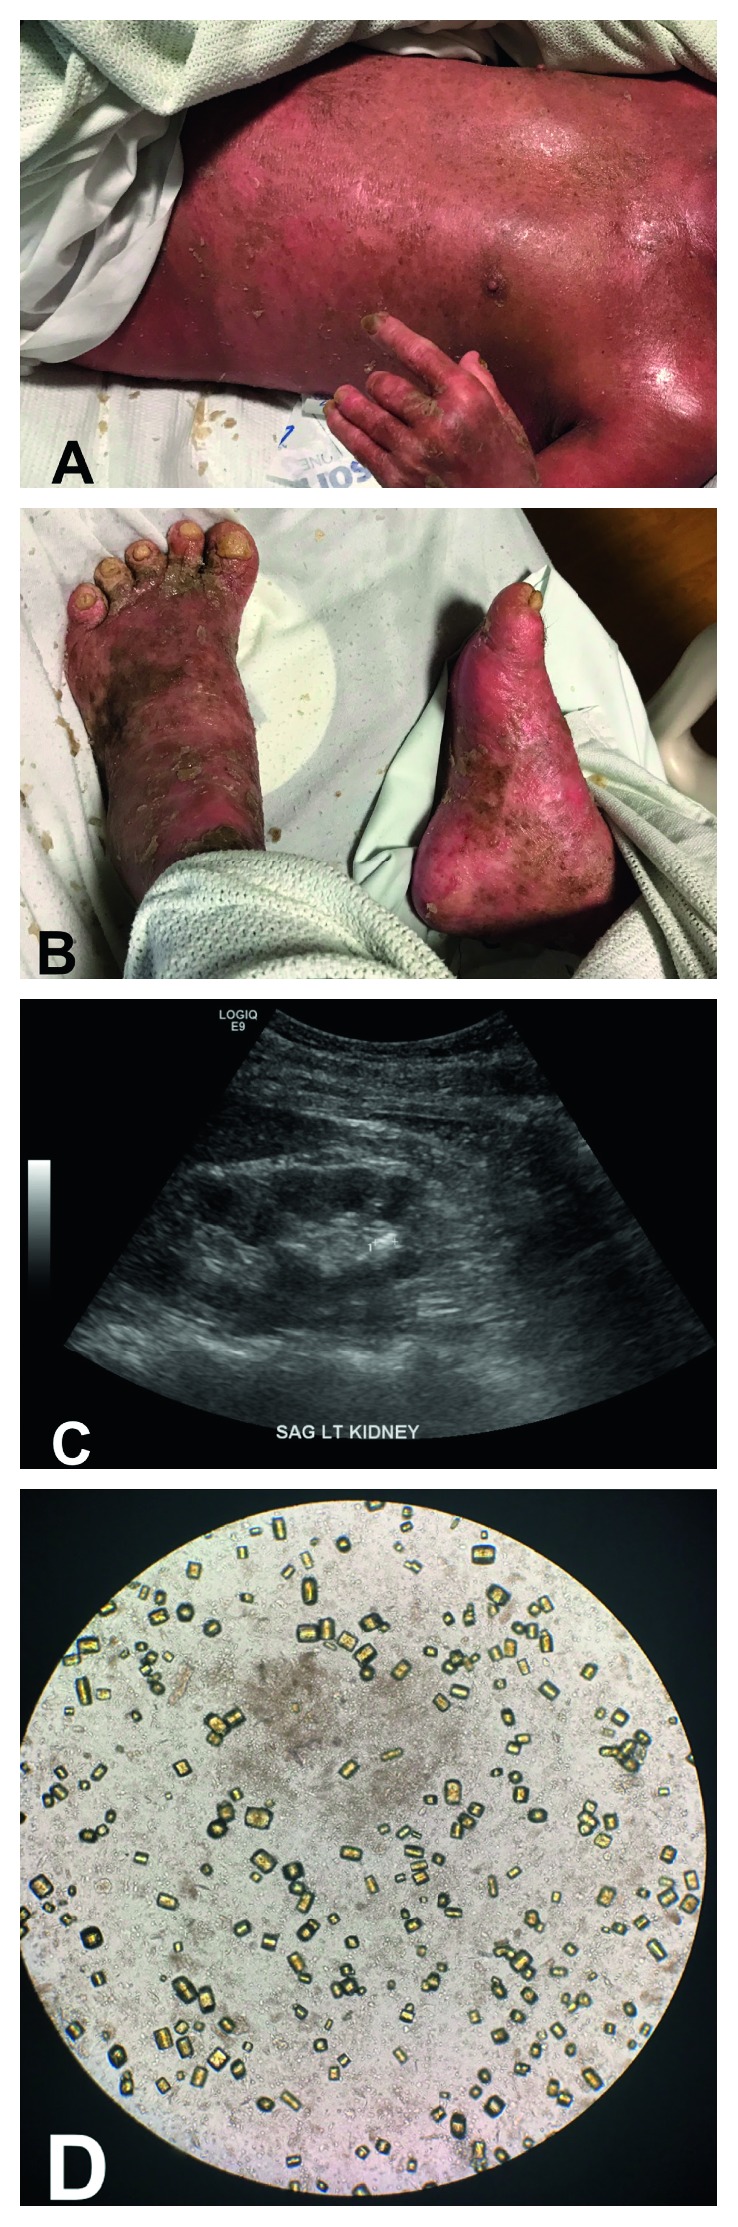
(a) The patient with exfoliative dermatitis with severe erythroderma on the upper torso. (b) Skin lesions also affecting lower extremities with nail involvement. (c) Renal ultrasound showing nephrolithiasis in the renal calyx. (d) Urine sediment displaying numerous rounded parallelogram‐shaped crystals, consistent with uric acid crystals.
